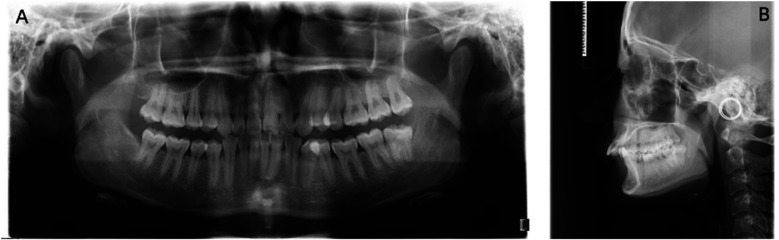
Radiological examination performed before orthodontic treatment. (A) Orthopantomogram. (B) Cephalometric radiograph.
